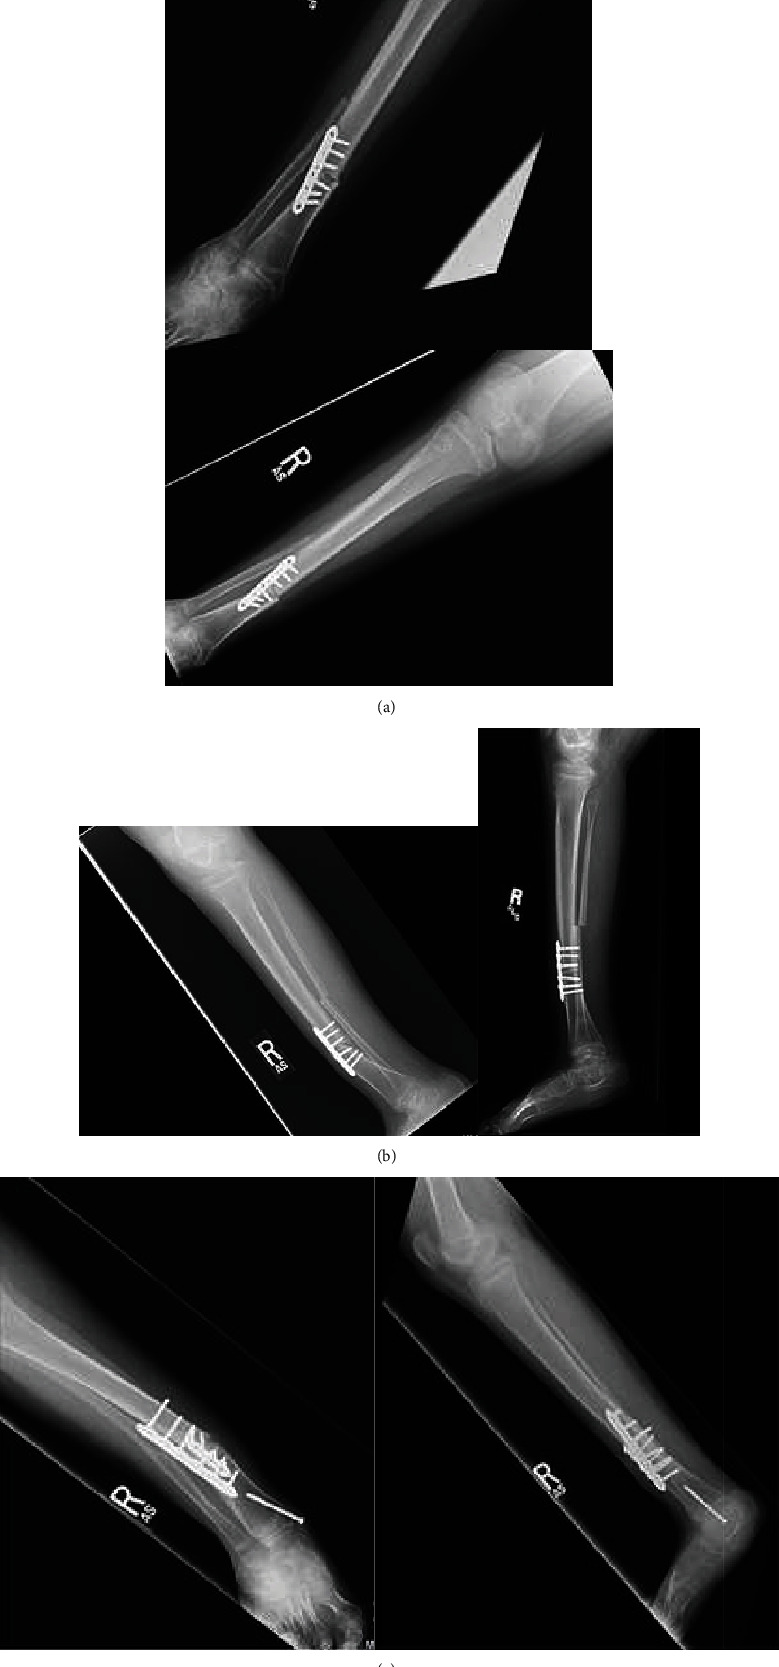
Supramalleolar derotational osteotomy in an 8-year-old male fixed with a 2.7 mm plate. (a) Postoperative anteroposterior and lateral radiographs. (b) Radiographs obtained at the 2-month postoperative visit demonstrating procurvatum and valgus angulation through the tibial osteotomy site. (c) Imaging obtained 14 weeks postrevision with perpendicular plating showing healing of osteotomy site with some residual valgus deformity.
